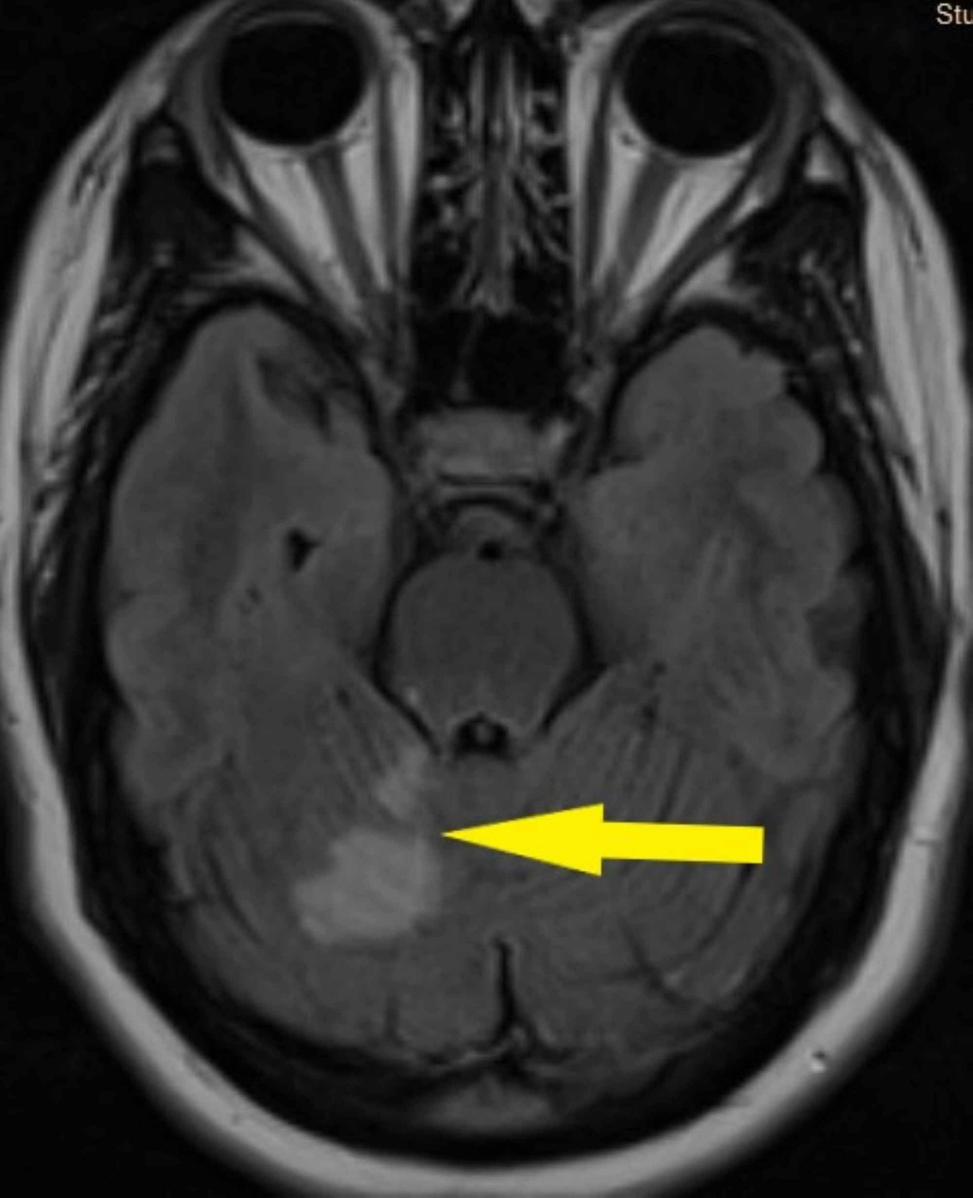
Magnetic resonance imaging of the brain (Flair) shows acute right cerebellar infarct
